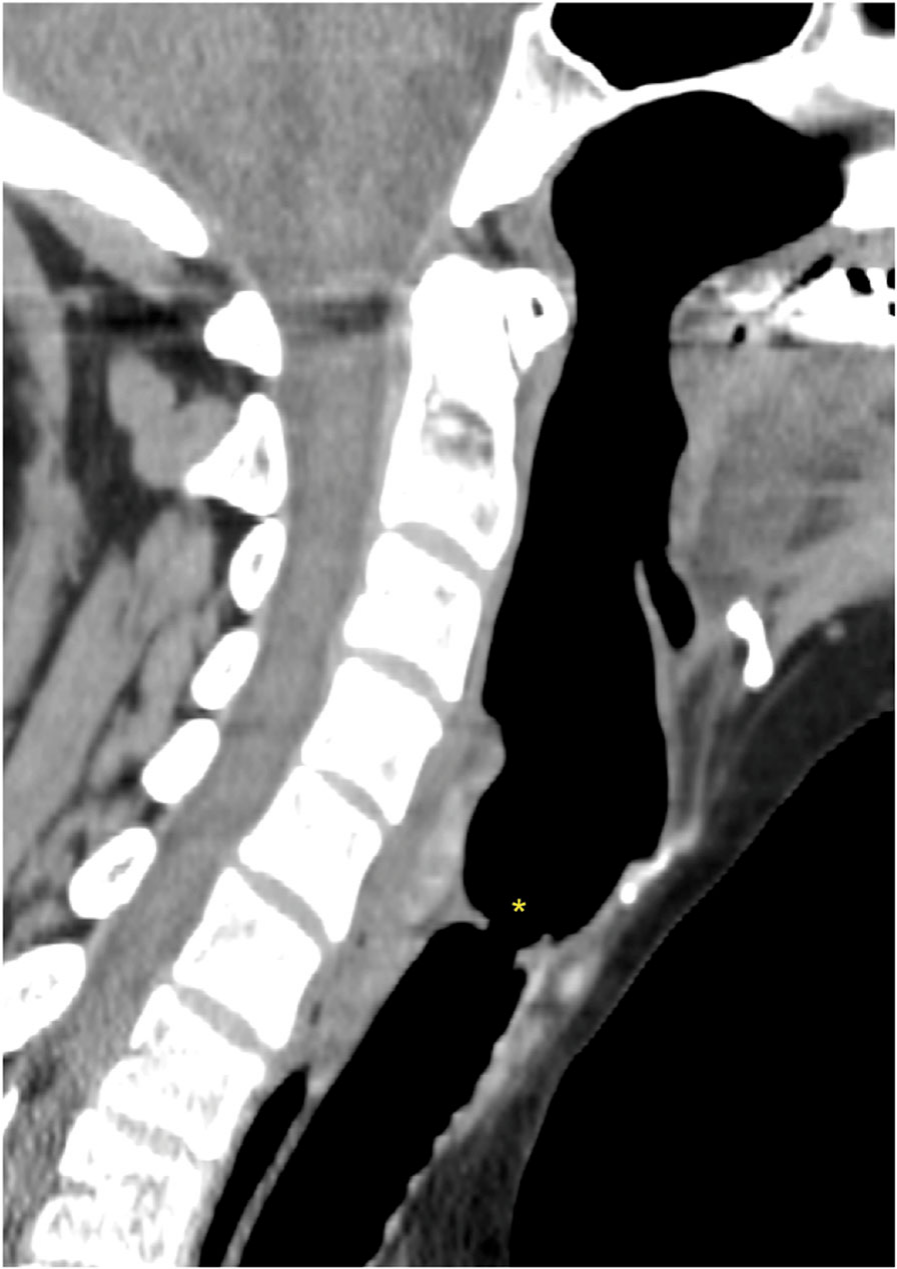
CT-scan longitudinal section of patient 2’s laryngotracheal stenosis (*)
